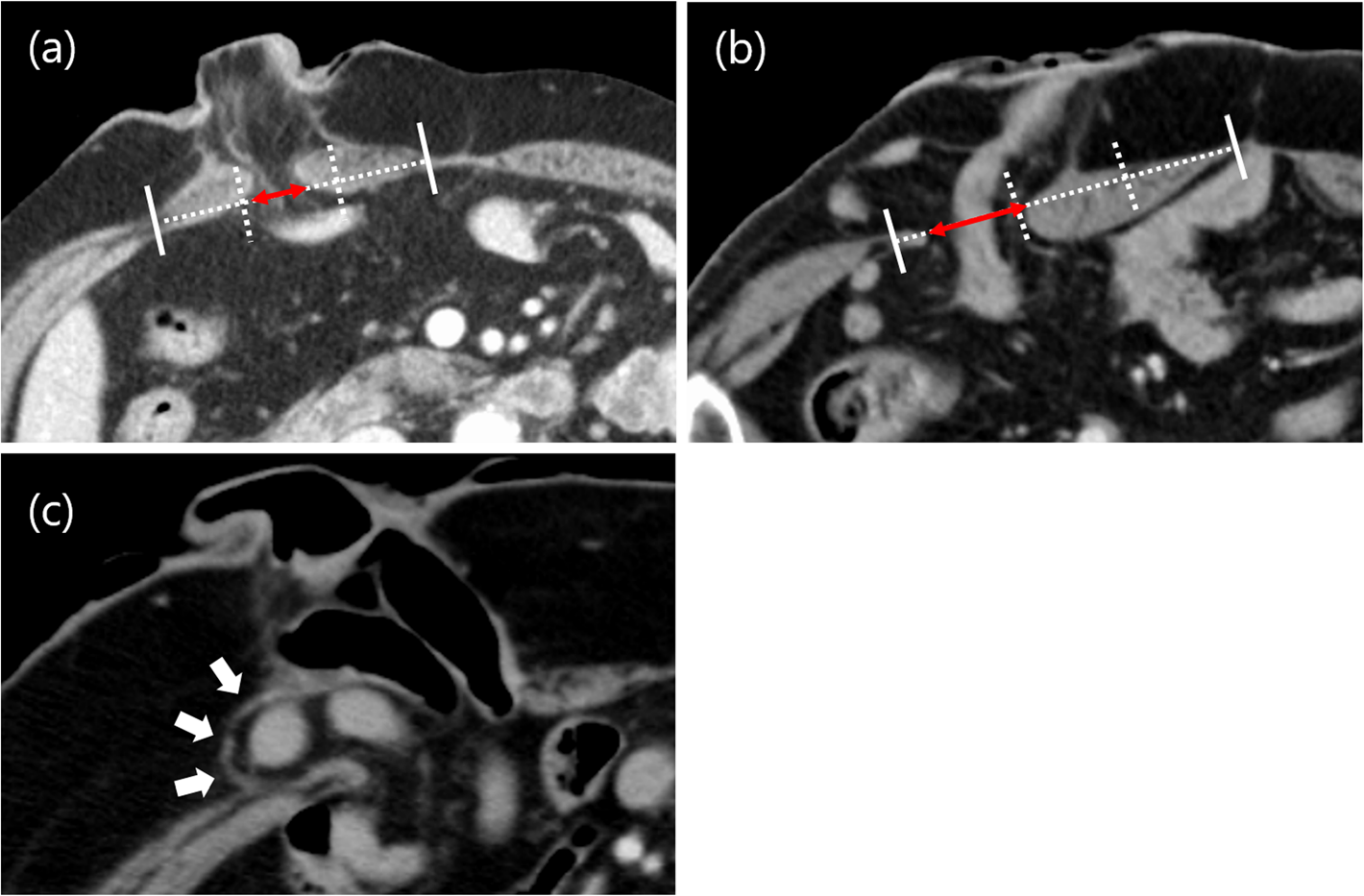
Definition of the stoma position and parastomal hernia. (a) A stoma passing through the middle of the rectus abdominis muscle. (b) A stoma not passing through the middle of the rectus abdominis muscle. (c) Postoperative abdominal CT was used to diagnose the parastomal hernia with a hernia sac (arrow)
